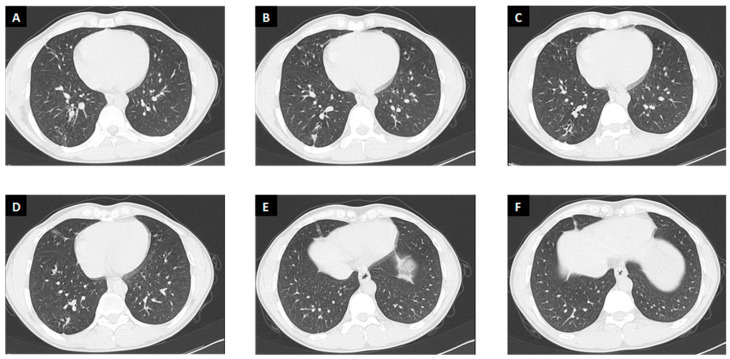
Chest CT scan at the end of treatment (Panel (A–F)).
